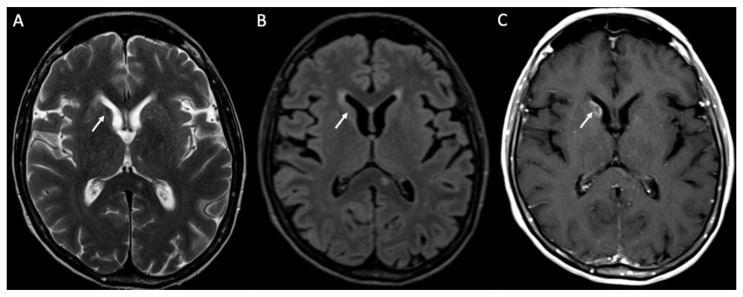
Axial T2 (A), FLAIR (B), and enhanced T1 (C) show a cryptococcoma in the head of the right caudate nucleus, which is slightly hypointense on T2 and FLAIR, and show inhomogeneous enhancement (arrows in A–C).
